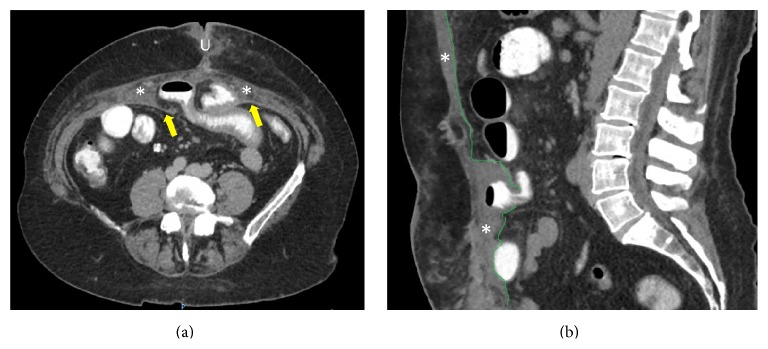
CT scan with intravenous and oral contrast. (a) Axial view. Defect in the posterior rectus sheath (arrows) with small bowel loops between parietal layers. (*∗*) Rectus abdominis muscle. U: umbilicus. (b) Sagittal view shows distension of proximal bowel loops consistent with a small bowel obstruction.
